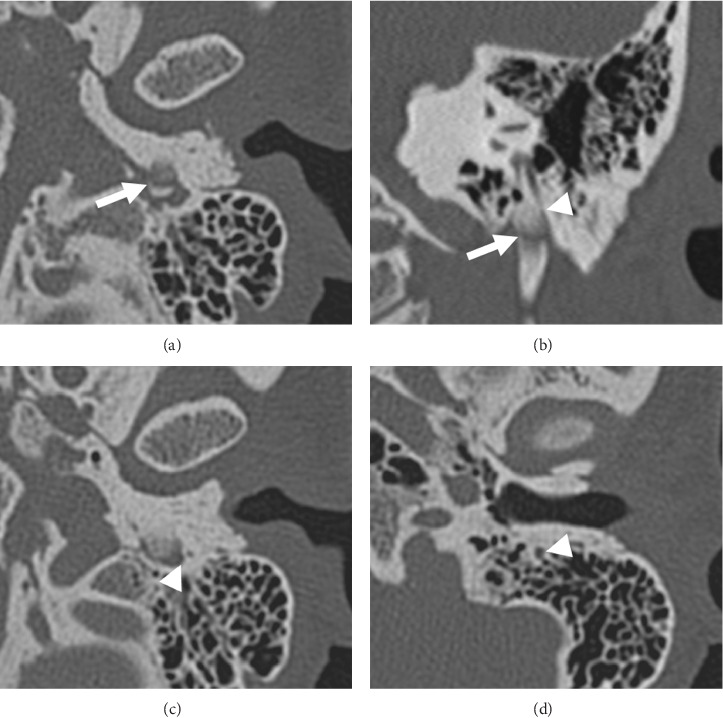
(a) Axial left temporal bone CT image at the level of the left stylomastoid foramen demonstrates an abnormal lucency suggestive of pseudoarthrosis through the base of the left styloid process (arrow). (b) Coronal left temporal bone CT image at the same level confirms the suspected pseudoarthrosis (arrow) and demonstrates apparent narrowing of the mastoid segment of the left facial nerve canal (arrowhead) due to osseous overgrowth of the base of the left styloid process at a level superior to the pseudoarthrosis. (c) Axial left temporal bone CT image at the level of the base of the left styloid process confirms narrowing of the distal mastoid segment of the left facial nerve canal (arrowhead) due to the overgrown left styloid process base. (d) For comparison, the axial left temporal bone CT image at the level of the left external auditory canal demonstrates the normal appearance of the mastoid segment of the left facial nerve canal (arrowhead).
